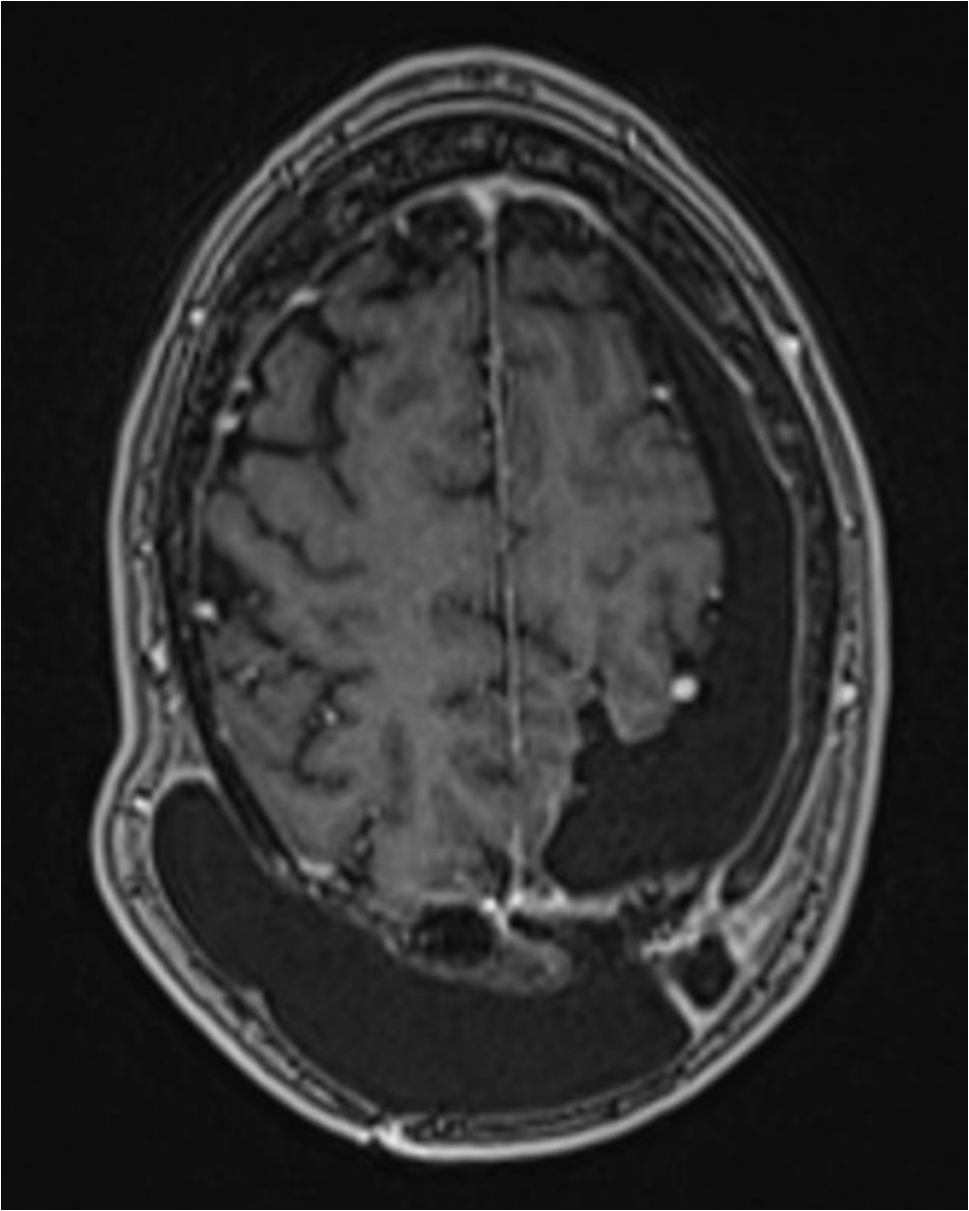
Preoperative axial T1-weighted MRI showing the extent of the subcutaneous CSF collection
